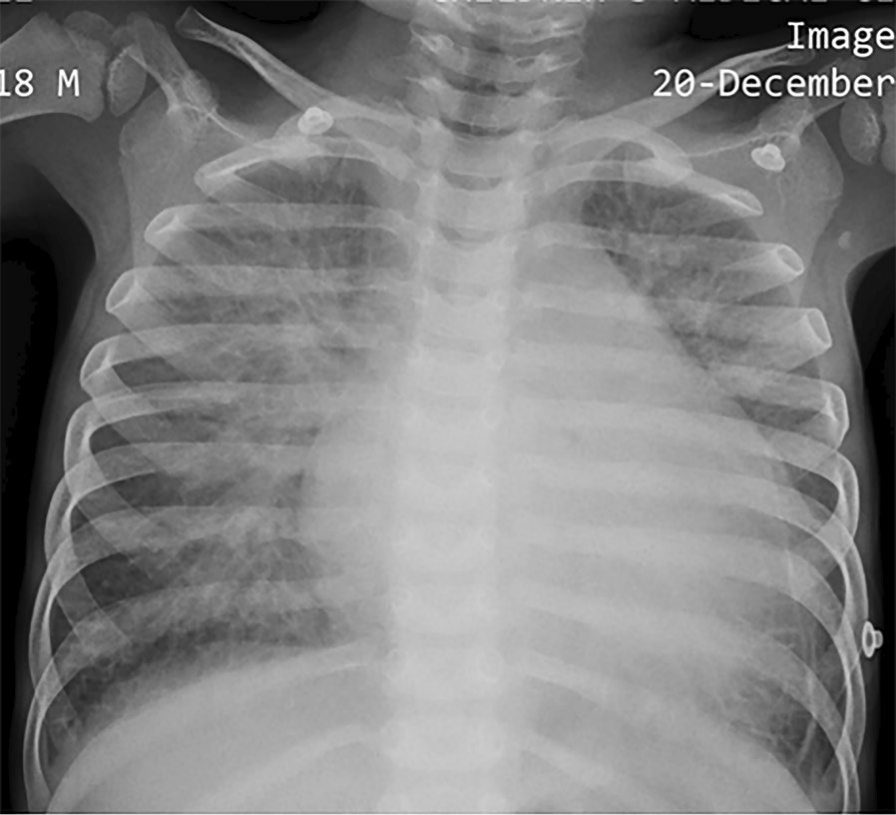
Chest X-ray of the patient showing cardiomegaly and pulmonary congestion
